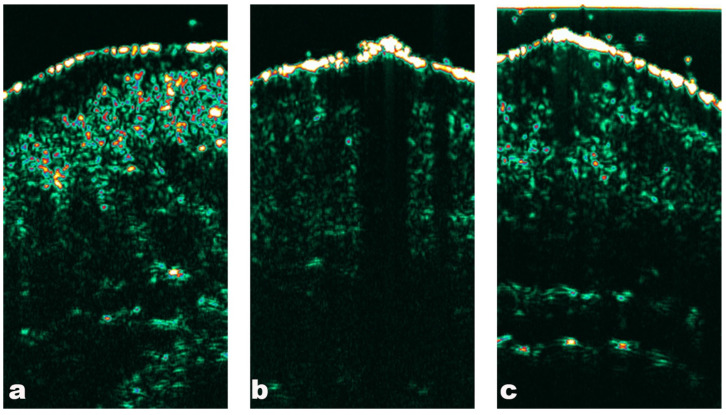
High-Frequency Ultrasonography (DermaScan C, 20 MHz) in actinic keratosis: (a) grade 1, (b) grade 2, (c) grade 3. Hypoechogenic structures underneath the entrance echo within the upper parts of the dermis; in higher grades, a perpendicular shadow corresponding to hyperkeratosis can be seen.
